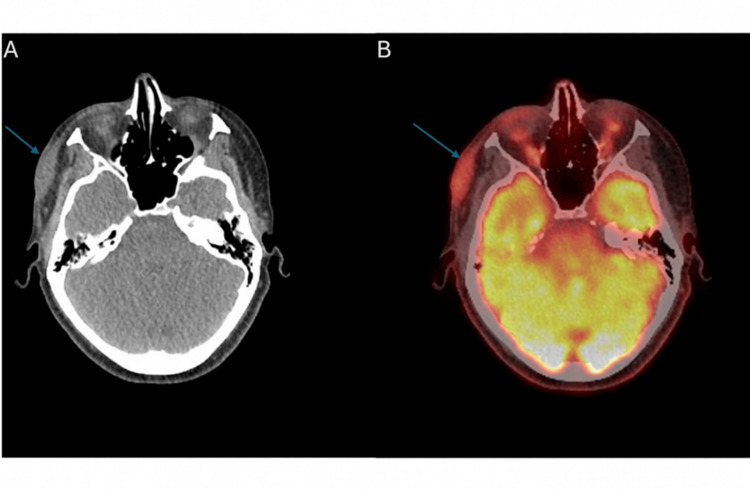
CT and PET-CT imaging. (A) Computed tomography demonstrating a 35 × 11 mm well-circumscribed, ovoid-shaped soft tissue thickening centered on the right temporal fascia just above the zygomatic arch (arrow). (B) Positron emission tomography-computed tomography (PET-CT) showing mild fluorodeoxyglucose (FDG) uptake within the same 35 × 11 mm well-circumscribed, ovoid-shaped soft tissue thickening centered on the right temporal fascia just above the zygomatic arch.
